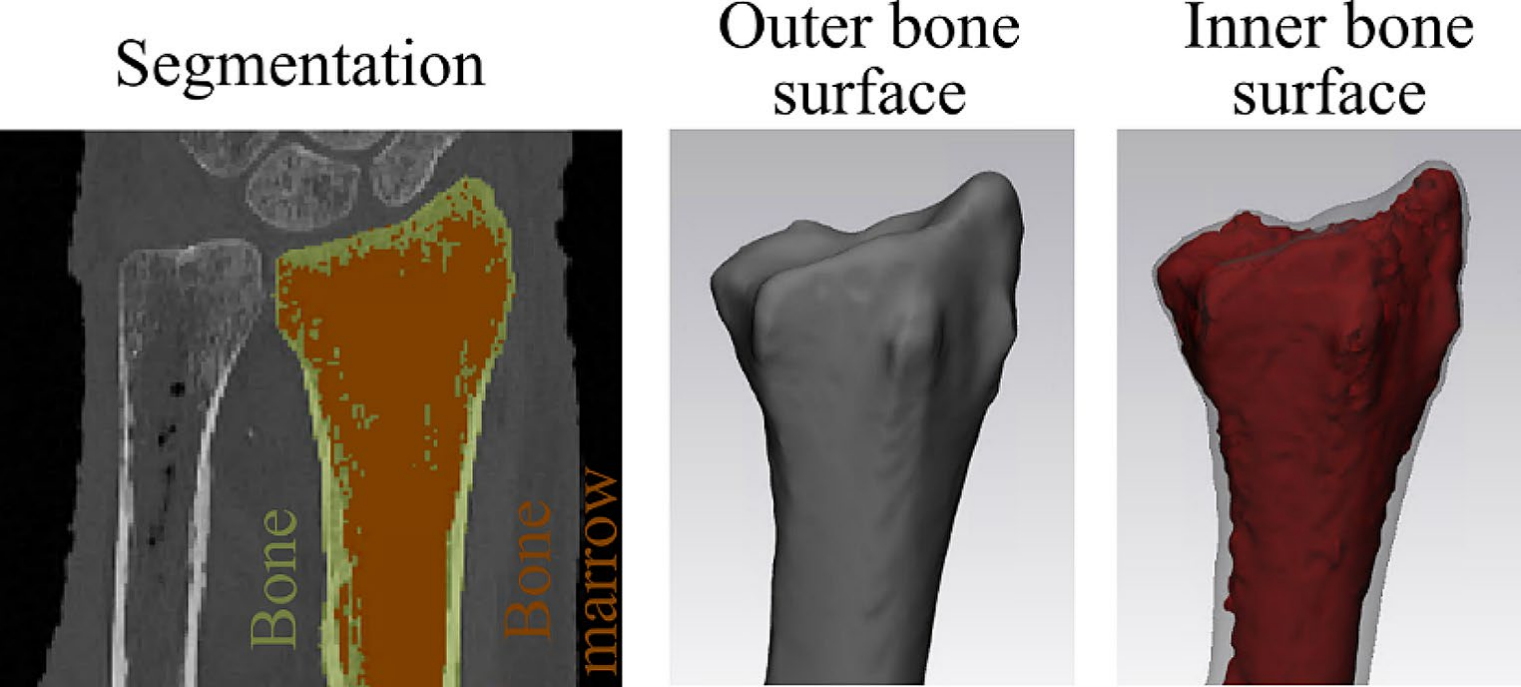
Image processing: CT image series were segmented with a manually determined threshold for the epiphysis and diaphysis and merged into a single bone mask. This mask was filled to obtain the outer bone surface. The inner bone surface was obtained as the subtraction of the filled and segmented bone mask
